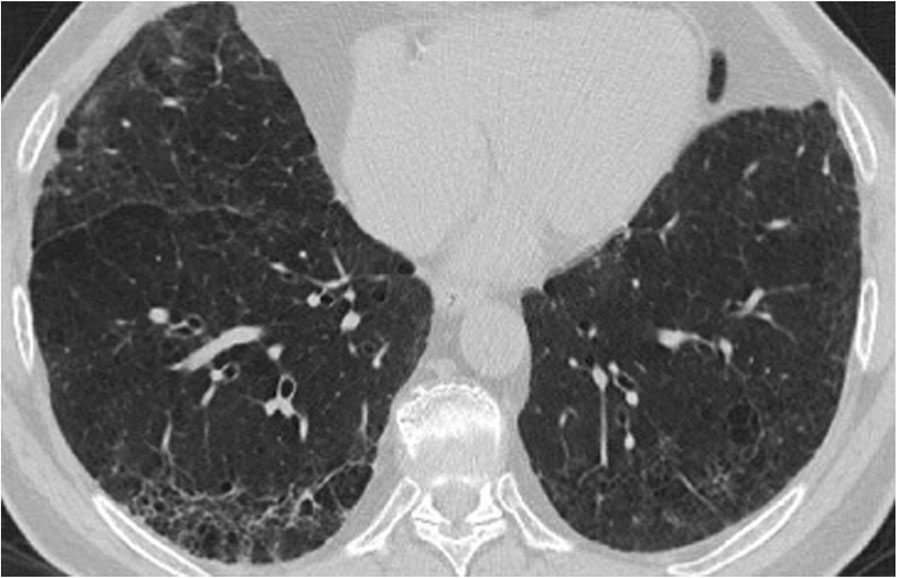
Chest HRCT showing a reticular pattern with peripheral traction bronchiectasis, with a predominant basal and sub-pleural distribution (probable UIP pattern)
